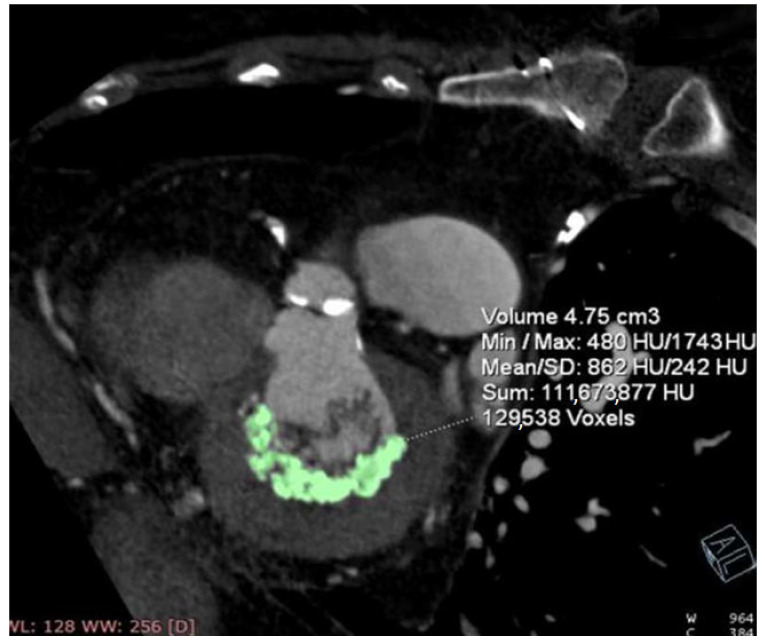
Contrast-enhanced cardiac computed tomography image demonstrating mitral annular calcification. The calcified mitral annulus is highlighted in green using a semiautomated segmentation overlay. Quantitative analysis of the segmented calcification volume and attenuation characteristics is displayed on the image.
